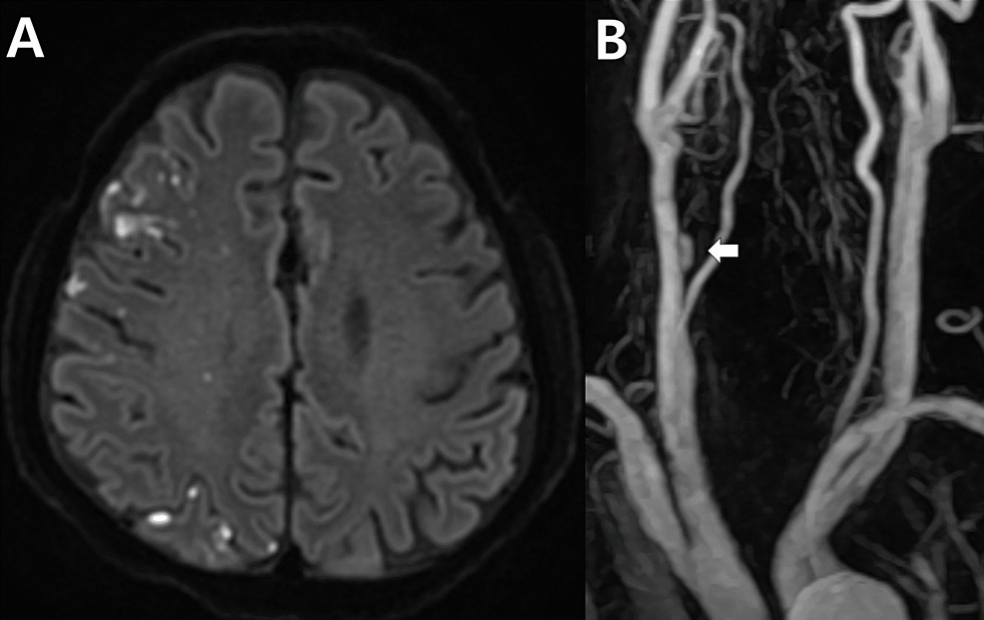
Follow-up radiological findings (A) Diffusion-weighted magnetic resonance imaging on the fourth day shows an increased number of multi-focal infarctions. (B) Neck magnetic resonance angiogram reveals a newly developed flow (white arrow) in the false lumen. Note the dynamic and unstable changes in the morphology of the mural hematoma when compared to the initial neck magnetic resonance angiogram.
